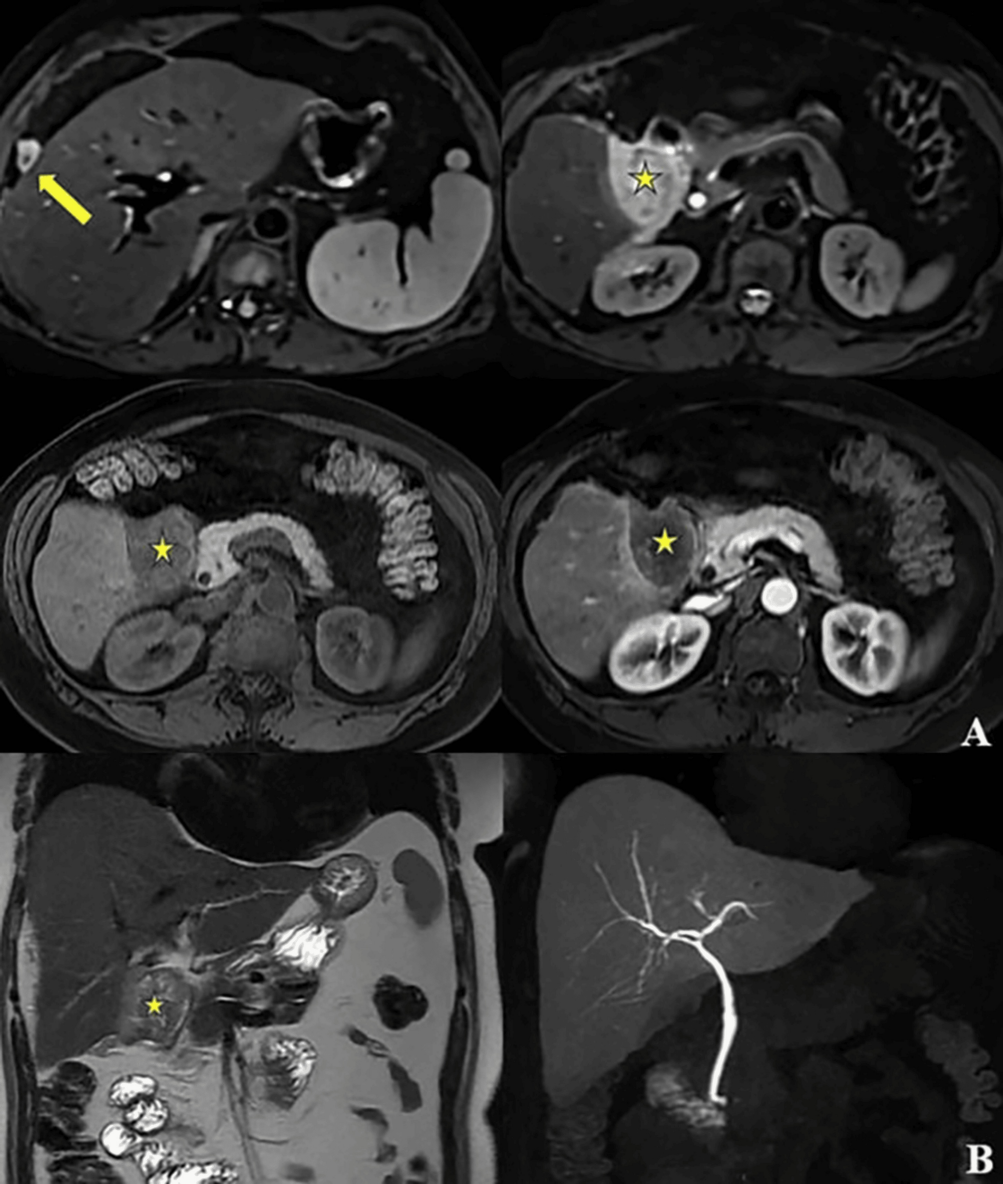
Magnetic resonance imaging revealing a 6.0 x 5.0 cm heterogeneous lesion between the right hepatic lobe and duodenum (star), with unclear margins and hypovascular pattern, featuring small nodules near the diaphragm (arrow). (A) Axial plane. (B) Coronal plane.
